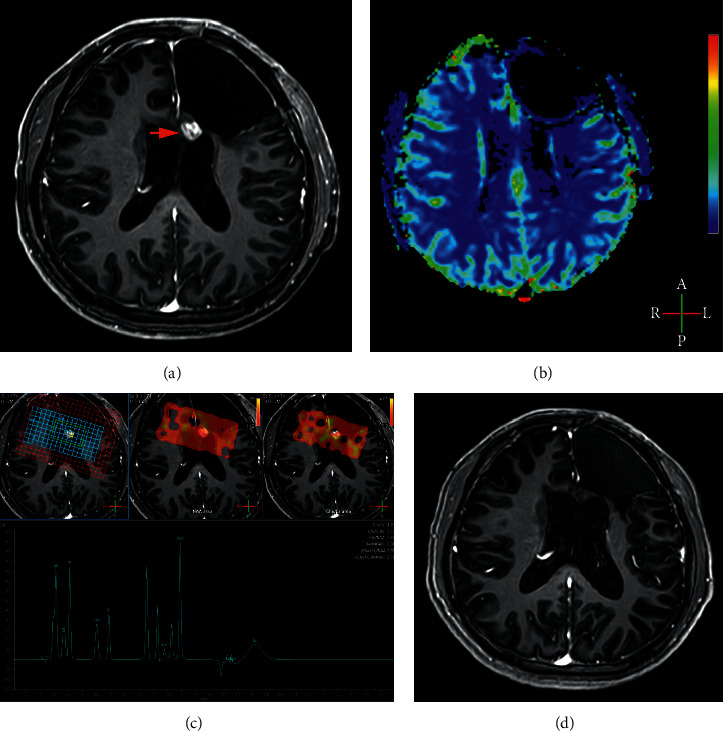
Example of pseudoprogression. A 21-year-old man with pathologically proven glioblastoma. A new enhancing lesion (arrow) developed in his left frontal lobe three months after completion of radiotherapy (a). No hyper-perfusion was detected on his DSC-MRI (b), with rCBV value of 0.71. MRS display Cho/Cr ratio of 1.20 and Cho/NAA 0.46 (c). The enhancing lesions in initial follow-up MRI disappeared in the MRI 23 months after completion of radiotherapy (d).
